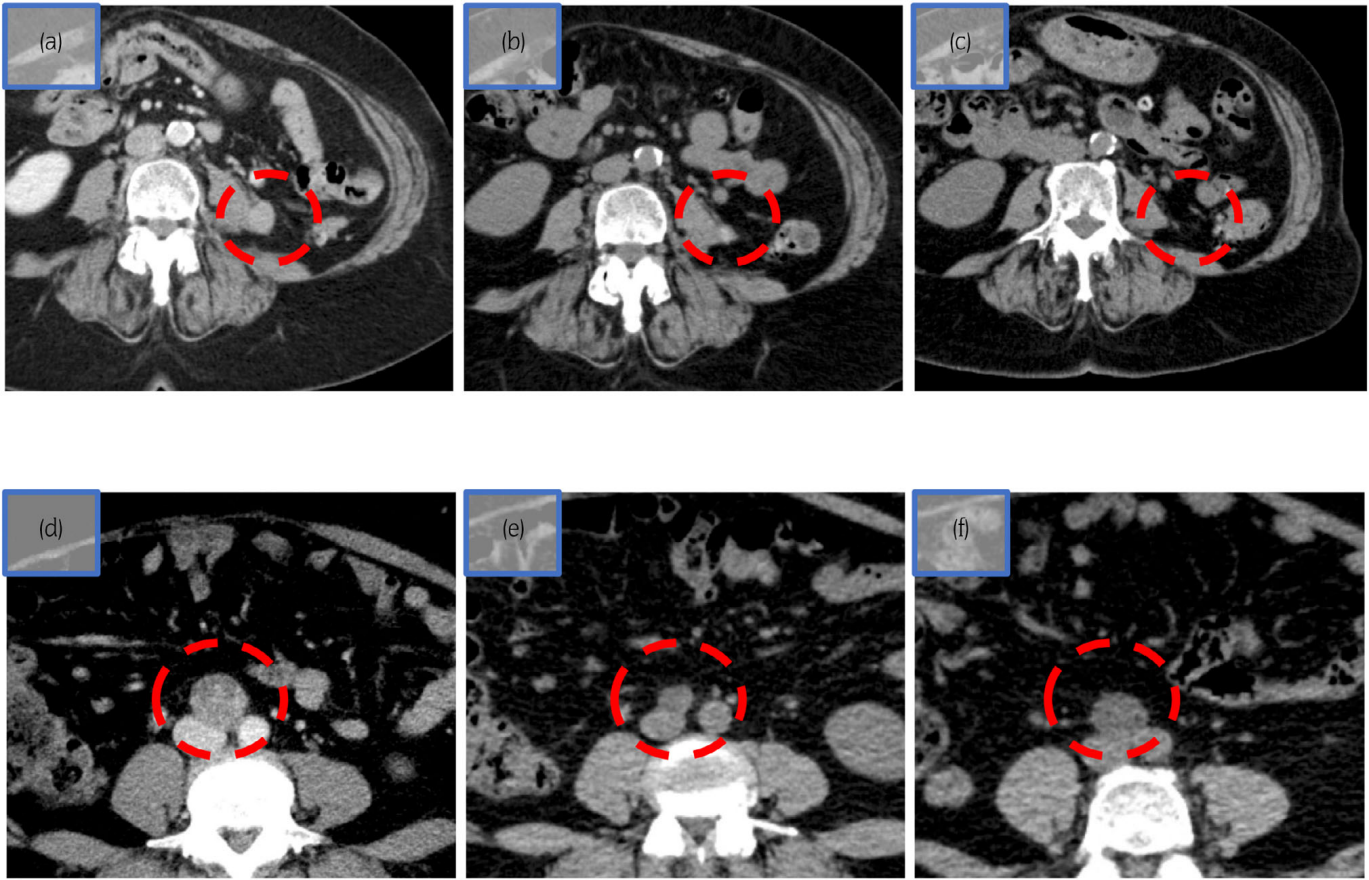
CT. (a–c) is first case and (d–e) is second case. (a) Twenty‐four months after surgery, retroperitoneal soft tissue tumor had a maximum diameter of 15 mm. (b) Thirty weeks after starting avelumab and axitinib, tumor size decreased to a maximum diameter of 10 mm, at 33% reduction rate. (c) Ninety weeks after starting avelumab and axitinib, tumor had almost disappeared (2 mm maximum diameter), at 86% reduction rate. (d) Para‐aortic lymph node with a maximum diameter of 32 mm. (e) Twenty‐five weeks after starting avelumab and axitinib, tumor size decreased to a maximum diameter of 13 mm, at 59%reduction rate. (f) Forty‐two weeks after starting avelumab and axitinib, tumor increased to a maximum diameter of 18 mm.
